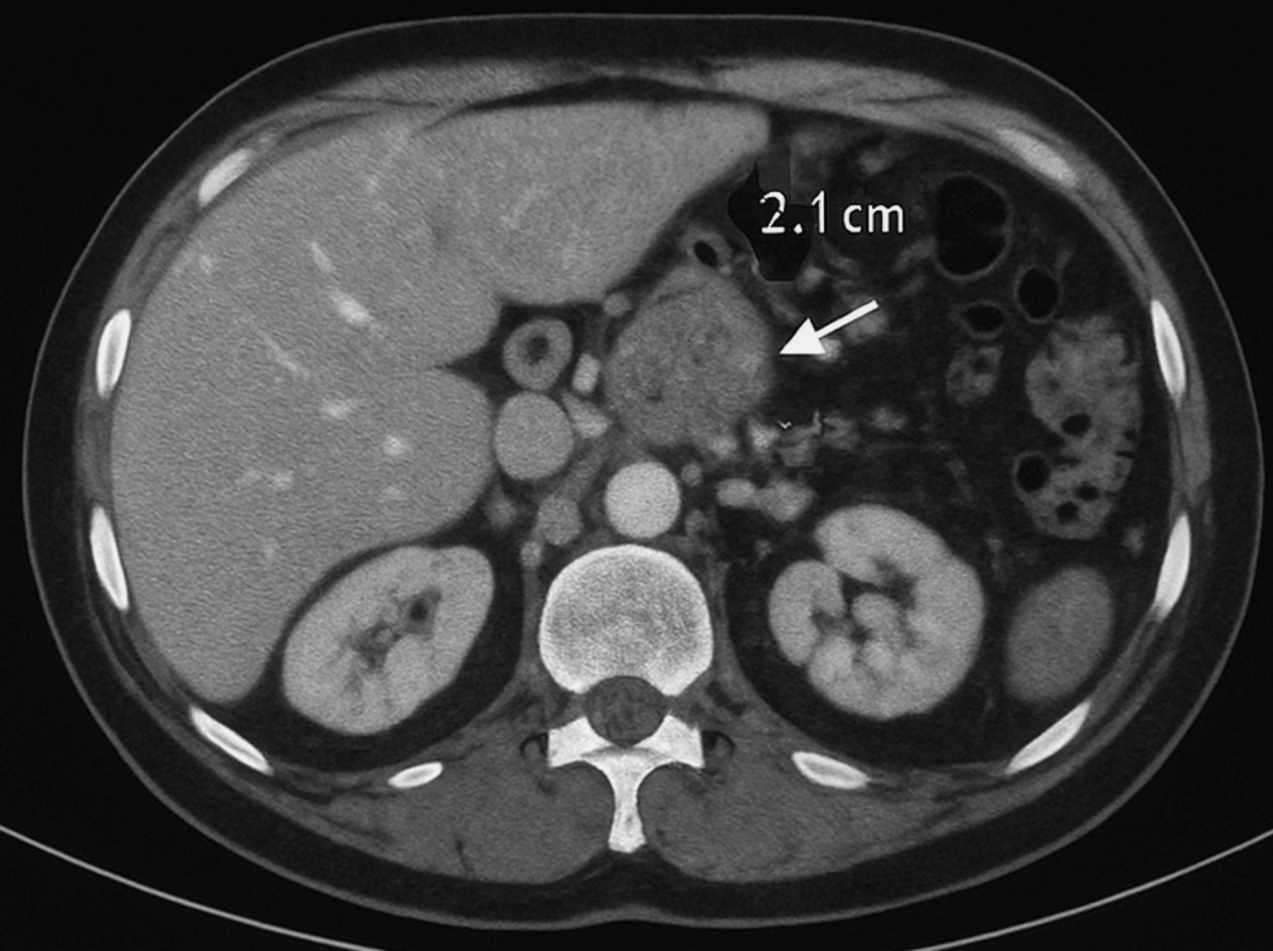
CT abdomen revealing soft tissue swelling in the head of the pancreas with peripancreatic lymphadenopathy The white arrow indicates the soft tissue swelling with its size of 2.1 cm. CT: computerized tomography
